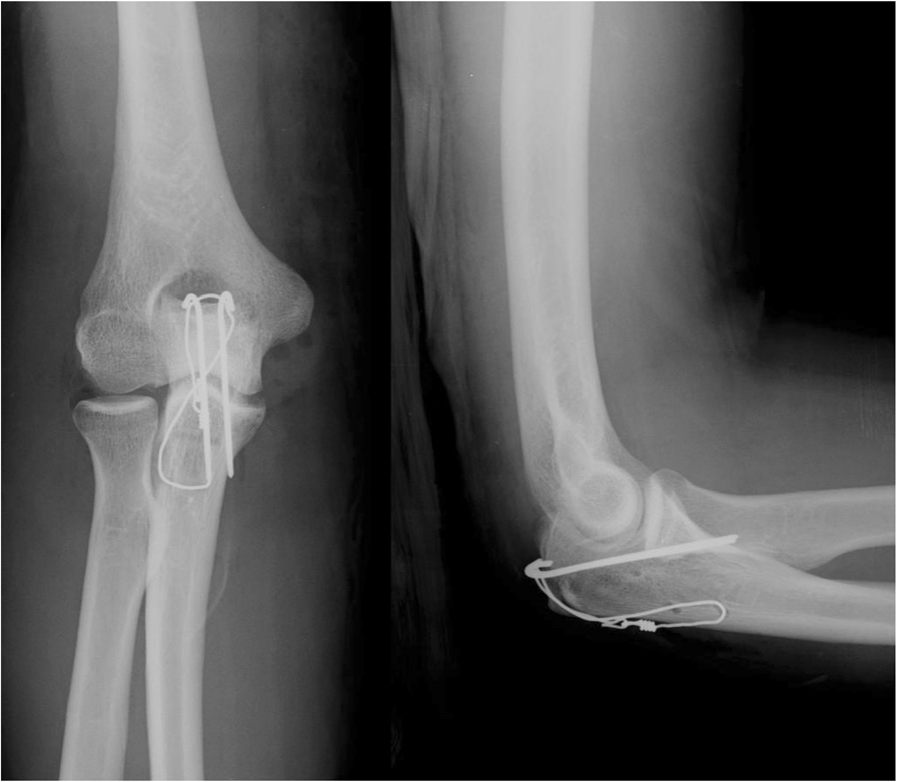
Postoperative radiographs showing the double drilling in the proximal part of the ulna. Olecranon avulsion fixed with a Zuggurtung tension band technique.
